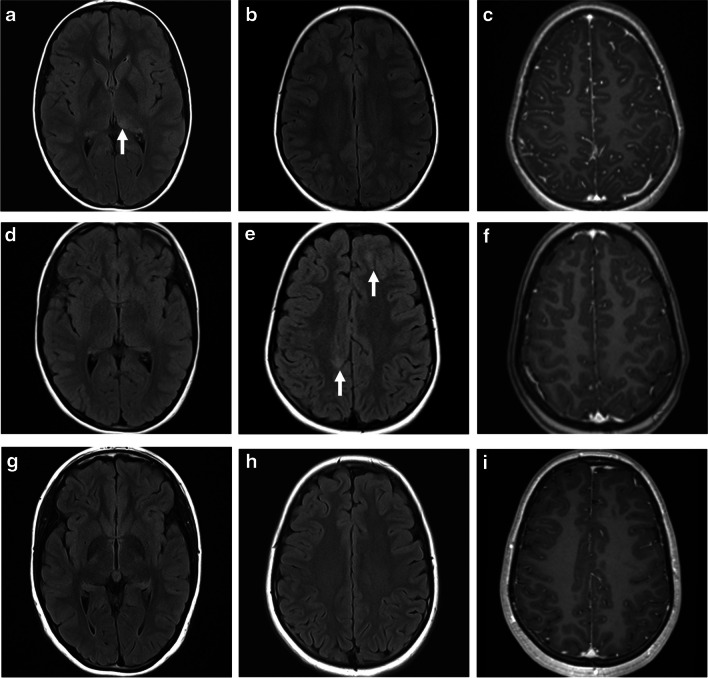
Lesion dynamics in myelin oligodendrocyte glycoprotein antibody-associated disease. a-c Magnetic resonance images of the brain obtained from an 11-year-old girl who presented with headache and vomiting, and was seropositive to myelin oligodendrocyte glycoprotein antibodies. a, b Axial fluid-attenuated inversion recovery (FLAIR) images show focal increased signal in the left thalamus (*arrow* in a), with diffuse sulcal effacement (b). c Axial contrast-enhanced T1-weighted image shows bilateral leptomeningeal enhancement. d-f MR images obtained at 3-month post-attack. d, e Axial FLAIR images show interval resolution of the signal abnormality in the left thalamus (d), with normal appearing sulci (e). f Axial contrast-enhanced T1-weighted image shows interval resolution of leptomeningeal enhancement. However, there are new subcortical increased FLAIR signal lesions (*arrows* in e) that are likely representing radiological lag phenomenon, as the patient was asymptomatic at that time. g, h Axial FLAIR and (i) axial contrast-enhanced T1-weighted images, obtained at 4-year post-attack, show normal appearance of the brain. This patient did not receive maintenance therapy
